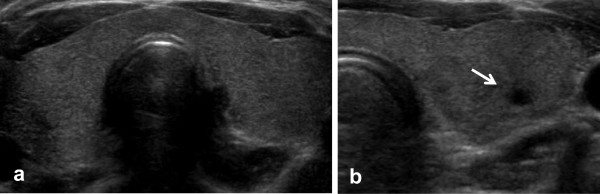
(a) The transverse thyroid sonogram shows a diffusely enlarged thyroid gland with isoechoic and non-coarsened background parenchyma. (b) A 0.4 × 0.3cm sized hypoechoic nodule (arrow) in the left thyroid.
